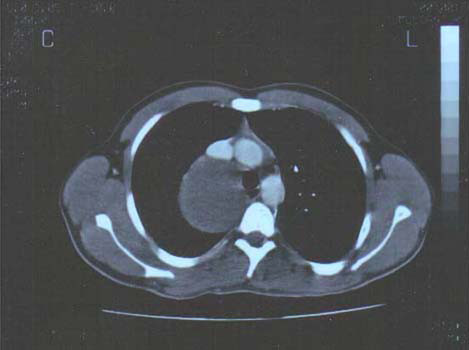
CT scan post contrast media injection reveals a huge low density compressive lesion in the posteromedial mediastinum. Notice the dislodgment of the thoracic vertebral body, the different dimensions of the spinal foramens and the different angles between the vertebral body and the traverse apophyses.
